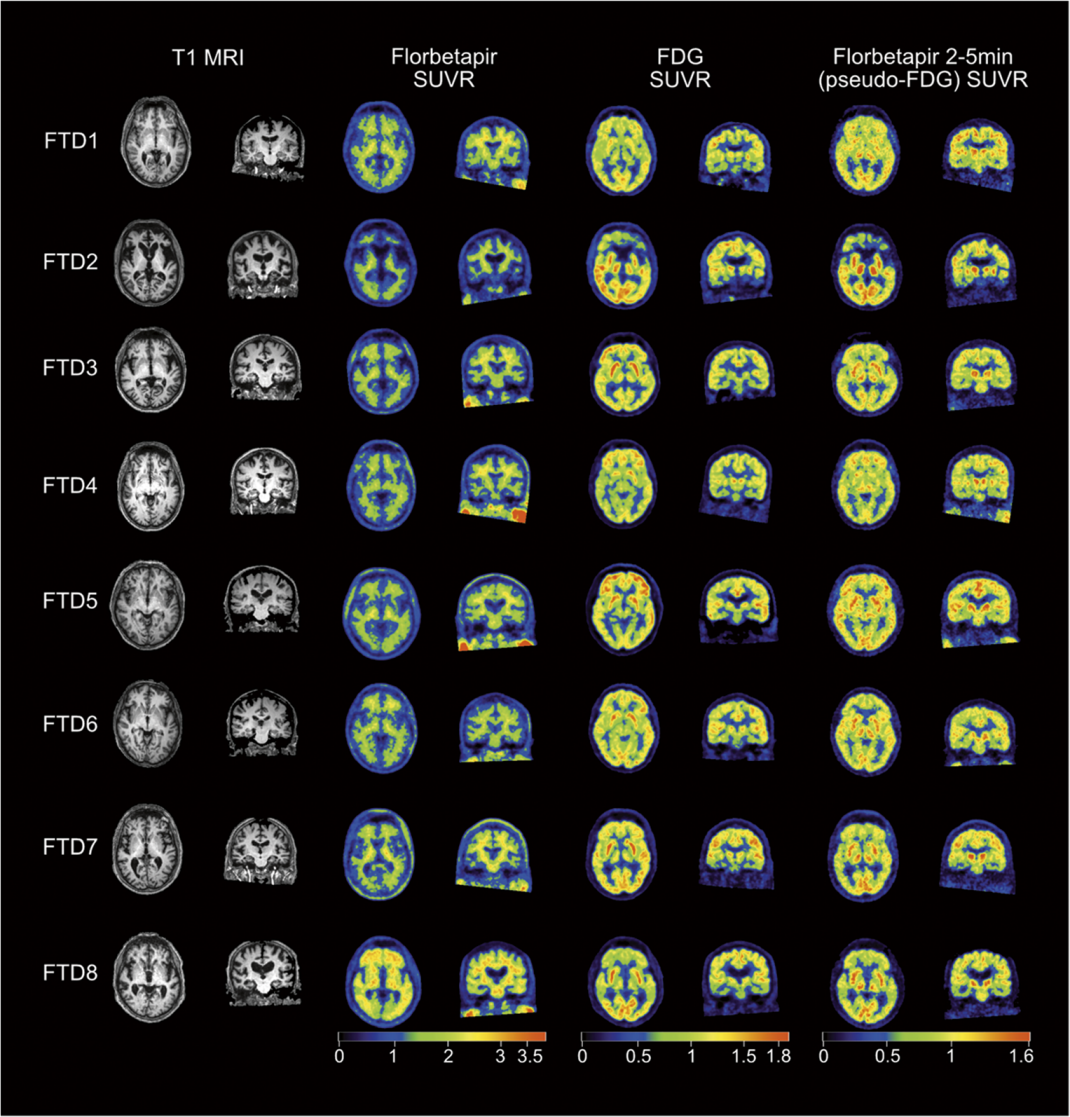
All imaging data from the bvFTD patients is displayed. Each row represents data from a single patient; each column represents coregistered images in axial and coronal slices for T1 MRI, florbetapir, FDG, and pseudo-FDG. The* Colour bars* represent SUVR values. A clear visual correspondence is seen in the hypometabolic patterns from the FDG images and the pseudo-FDG images
